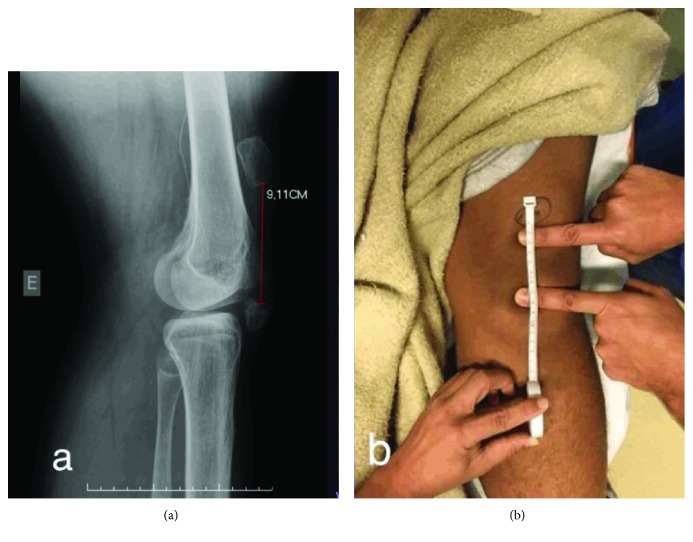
(a) Preoperative radiograph. (b) Preoperative clinical examination.
